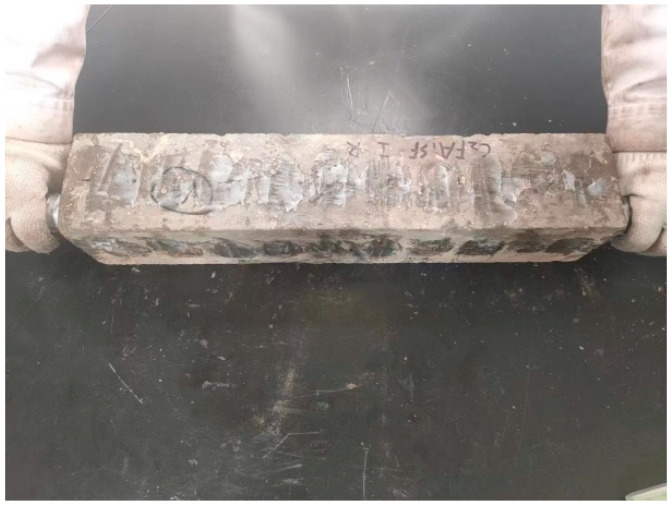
Ultrasonic Velocity Measurement.
